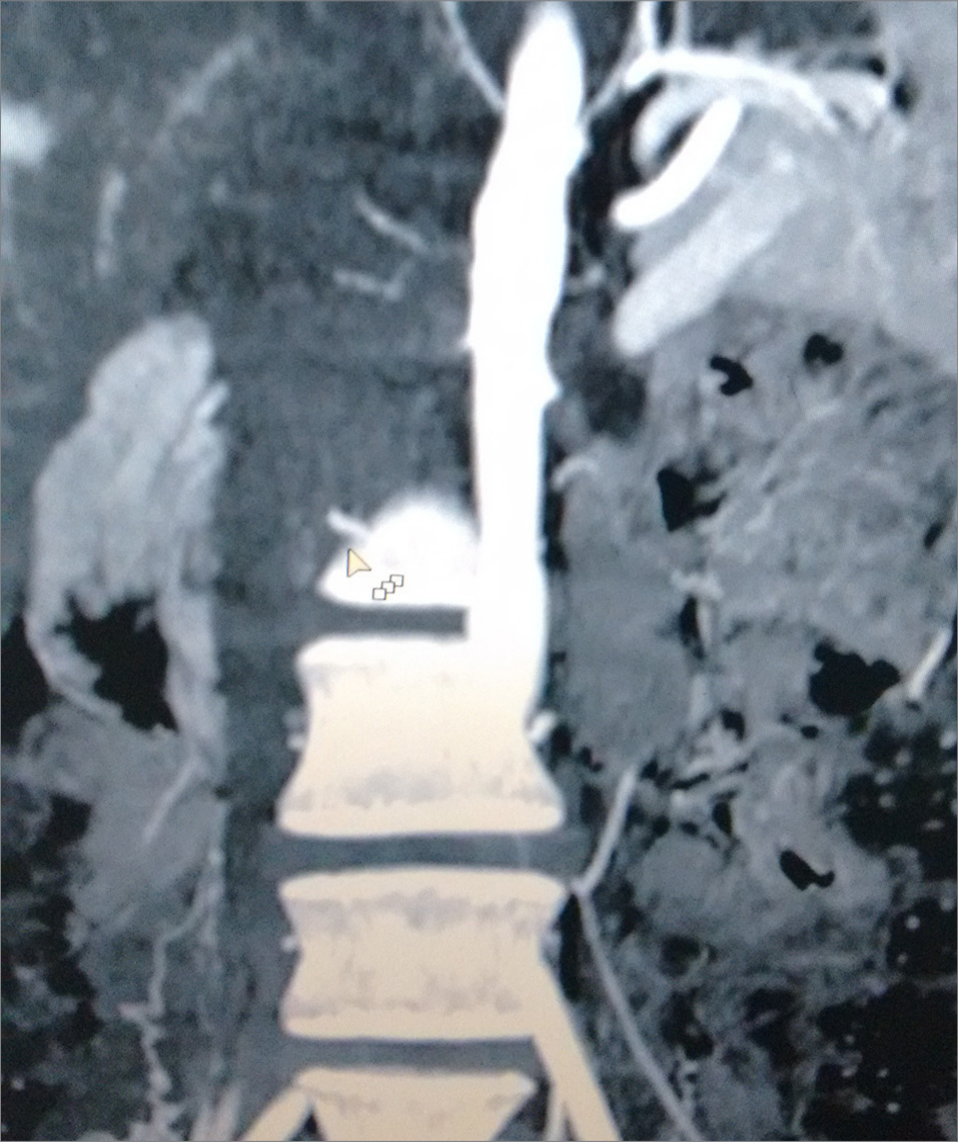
Multi-slice computed tomography angiography of the abdominal aorta showing absence of flow in the renal arteries.
